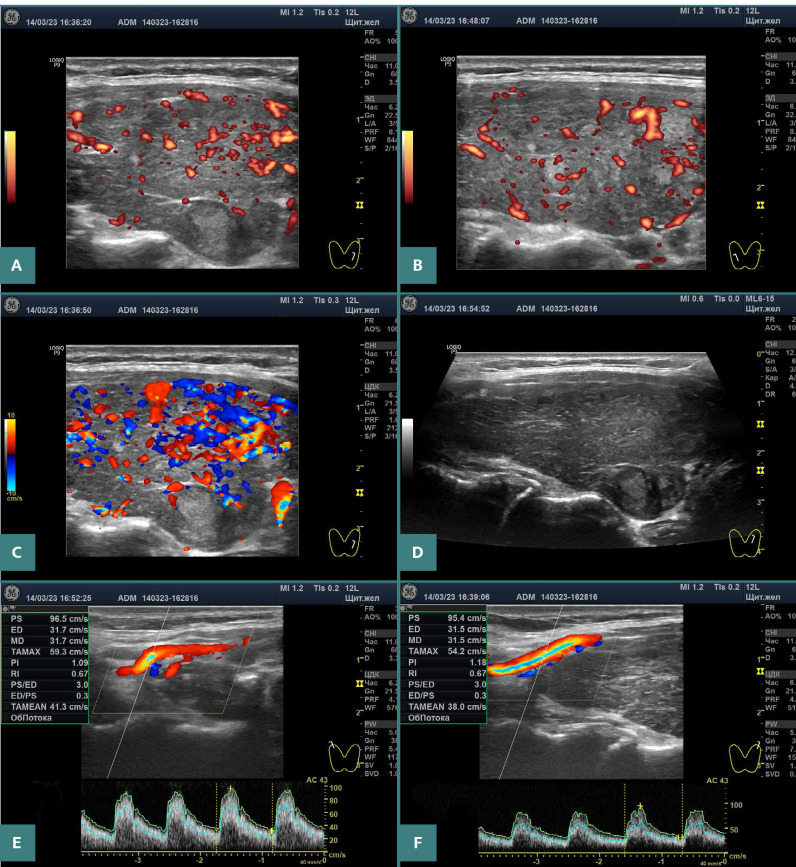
Ultrasound images of the thyroid of patient 2. A,B, Moderate increase in blood flow intensity in two lobes in power Doppler mode at optimal PRF. C, ‘Thyroid inferno’ sign in the left lobe in color Doppler mode at low PRF. D, Left lobe in grayscale mode. E,F, PSV in the first branch of the STAs: 96.5 cm/s on the right and 95.4 cm/s on the left.
